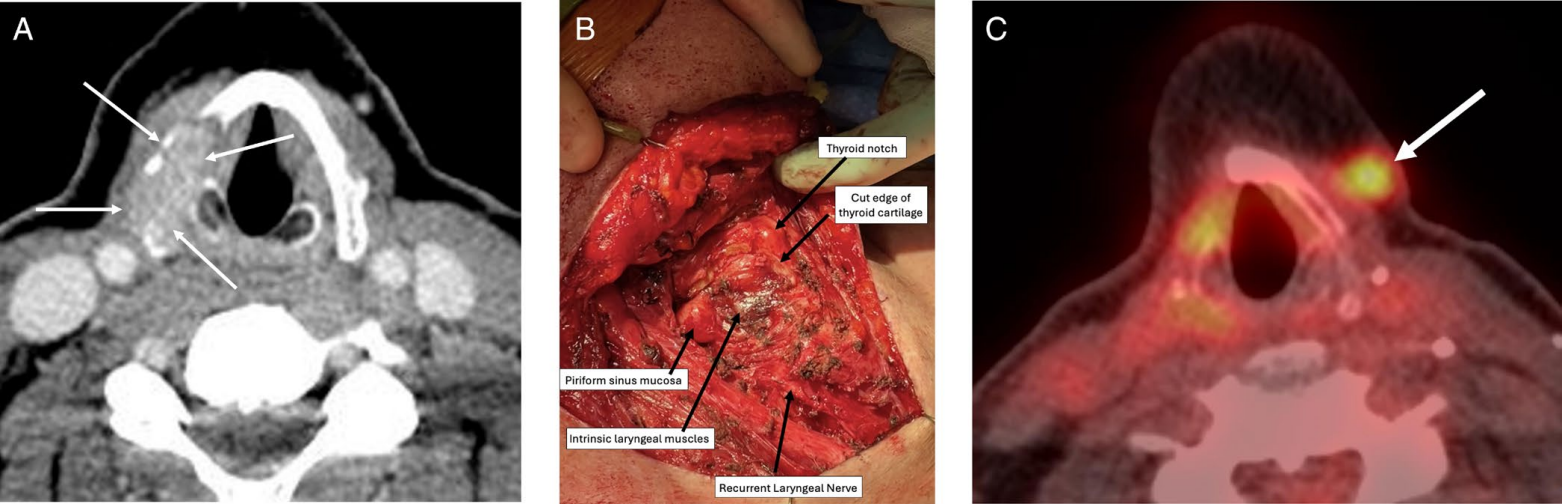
(A) Preoperative CT neck with contrast showing a 2.4 cm tumor (arrow) involving the right thyroid cartilage and extending into the paraglottic space. (B) Intraoperative photograph after right partial laryngectomy demonstrating laryngeal defect, with intrinsic muscles of the larynx, pharyngeal sinus mucosa, and intact recurrent laryngeal nerve. (C) Postoperative FDG PET CT scan 12 months after surgery showing the right thyroid cartilage defect, without evidence of laryngeal disease, but with a new 0.7 cm FDG avid focus of soft tissue disease superficial to the contralateral (left) sternohyoid muscle.
